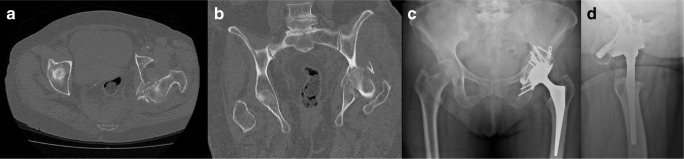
Pre-operative axial (a) and coronal (b) views on CT scan of an 82-year-old female patient who sustained a comminuted posterior wall fracture associated with posterior hip dislocation treated by DM-CHP with posterior approach only (SA-CHP). Post-operative anteroposterior pelvic (c) and axial hip (d) X-rays at 12 months of follow-up post-SA-CHP.
